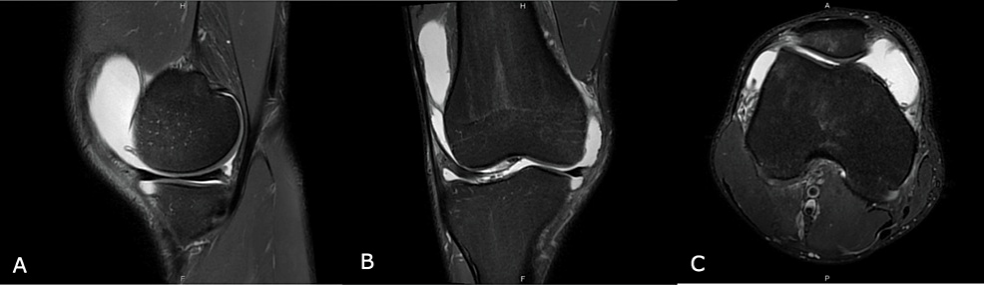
MRI of the right knee: T2-weighted sequence showing marked effusion with diffuse synovitis. (A) Sagittal plane. (B) Coronal plane. (C) Axial plane.
